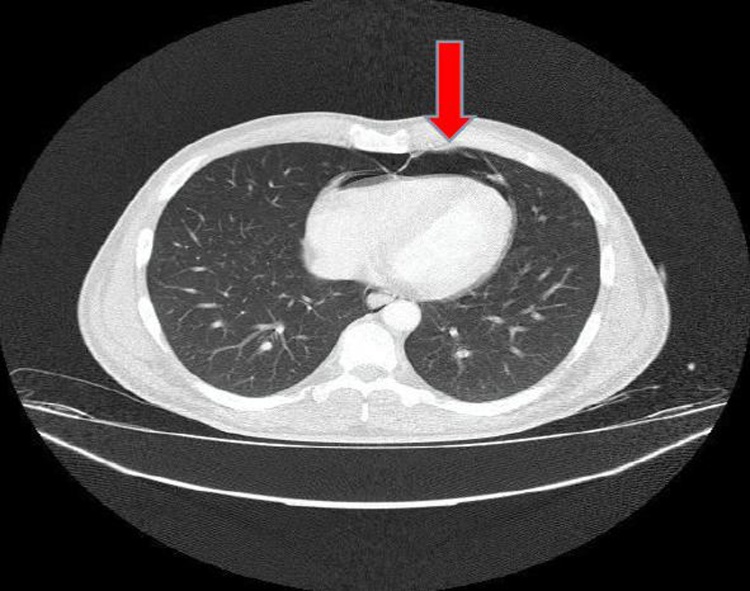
Pneumopericardium on Chest-CT.
